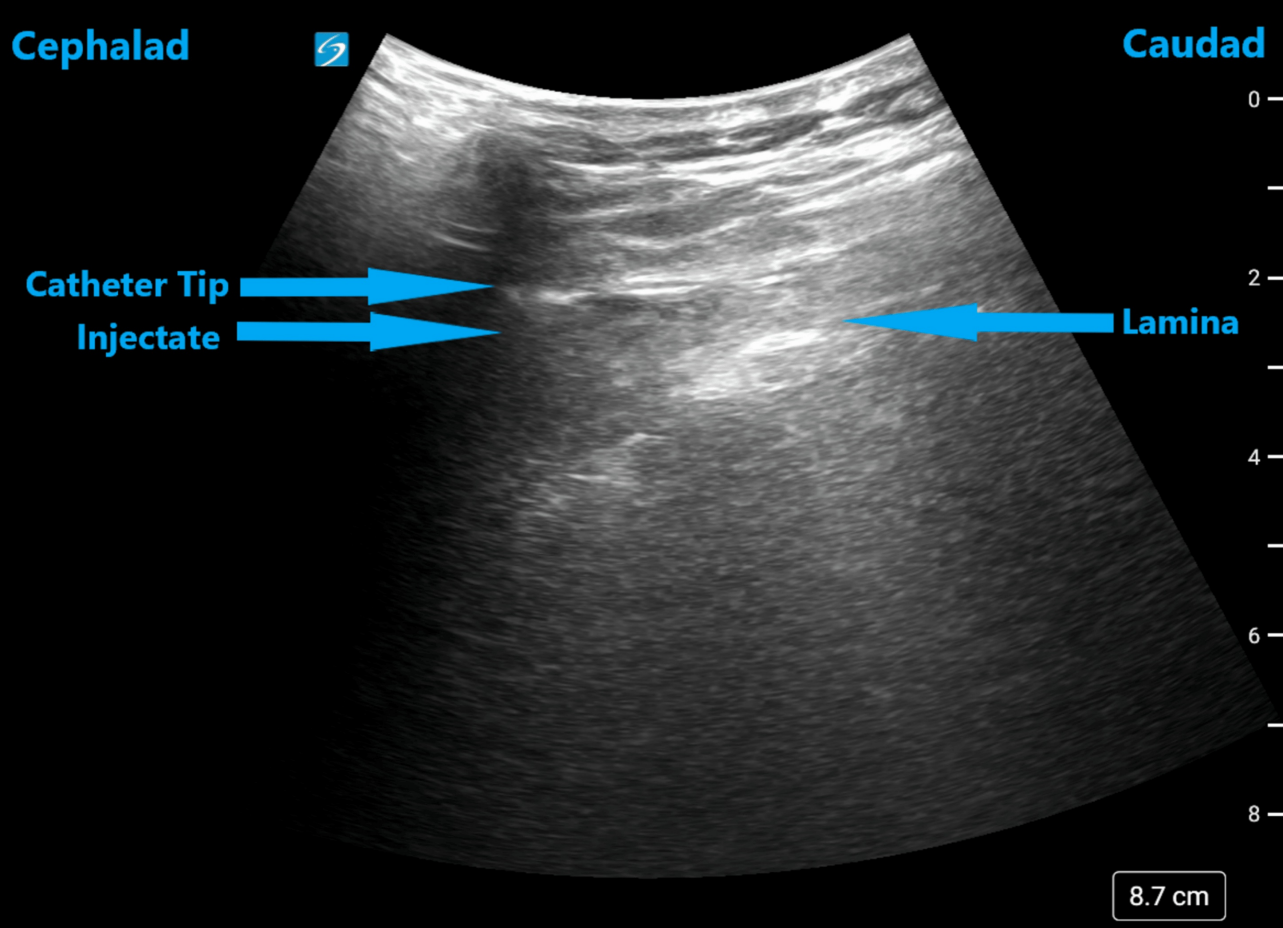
Ultrasound image of the thoracic spine in the parasagittal oblique view demonstrating the catheter outside the epidural space
